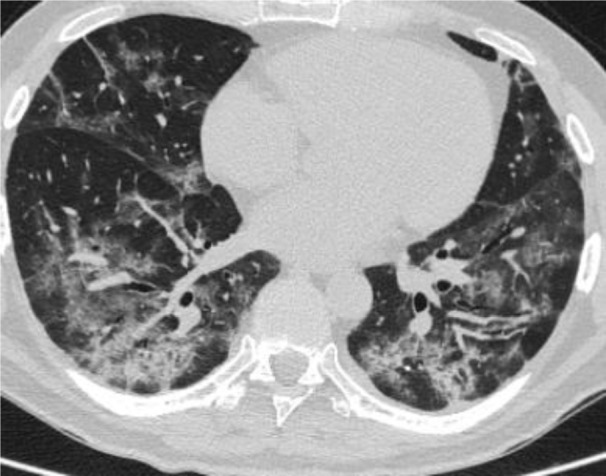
Chest MS CT scan after hospital discharge before xenon-oxygen gas mixture treatment
